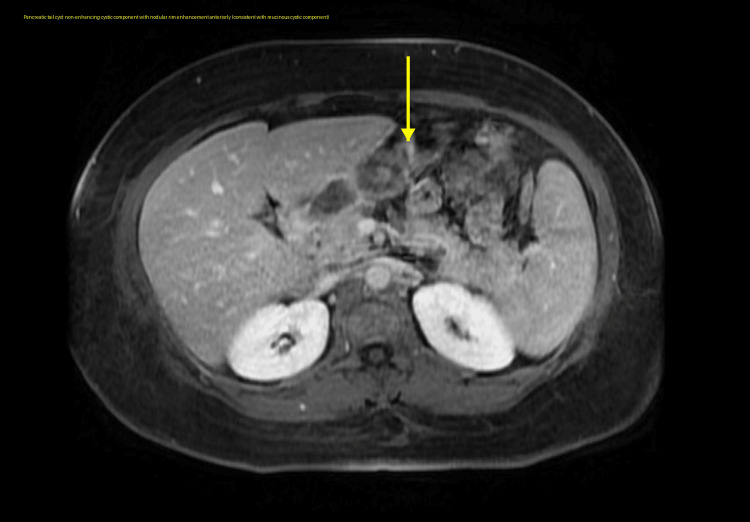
Axial post-contrast MRI demonstrating a pancreatic tail cyst with a non-enhancing cystic component and nodular rim enhancement anteriorly, consistent with a mucinous cystic component
